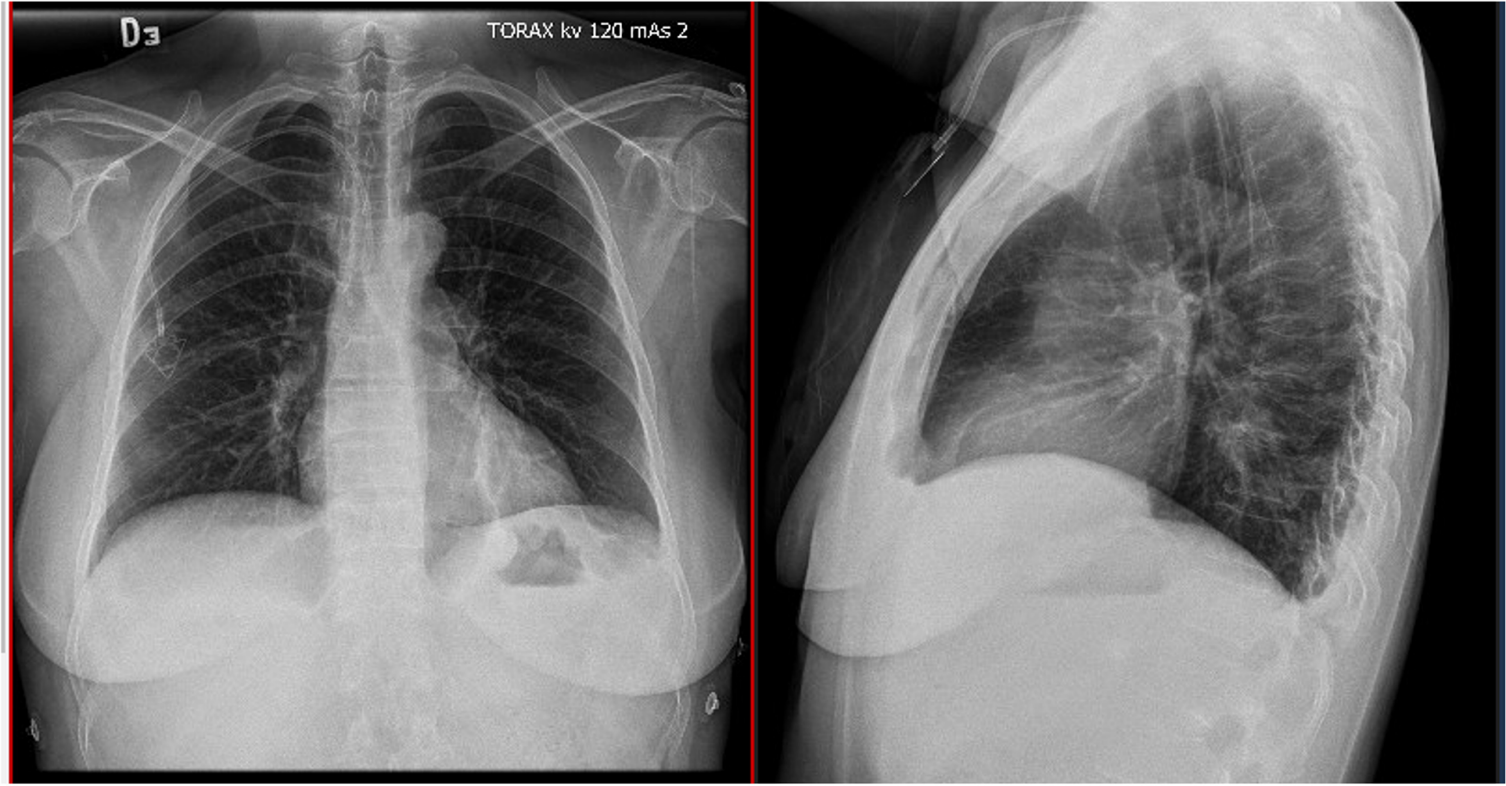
Thoracic x-ray with catheter with an arterial route.
